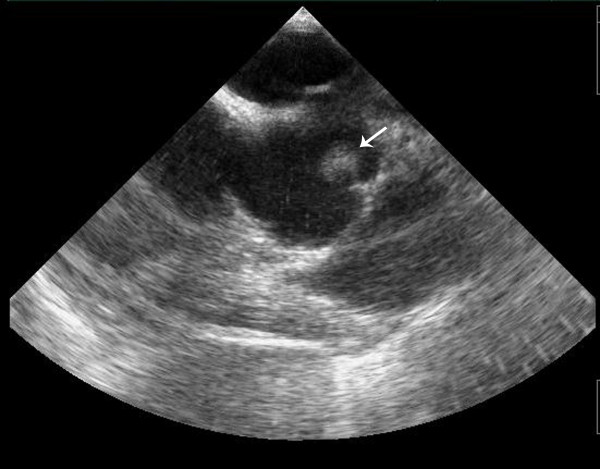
Trans-thoracic echocardiography demonstrating mobile mass on the left coronary cusp of the aortic valve.
